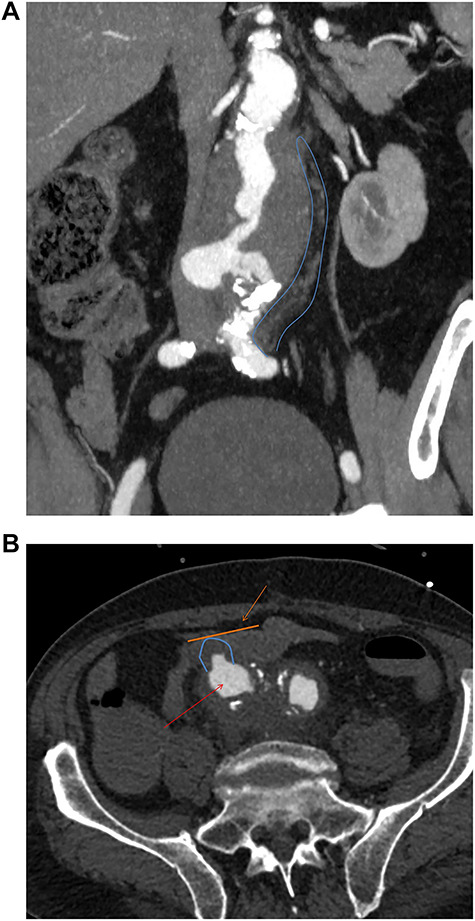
(a) Preoperative coronal thick slice of a CT with IV contrast in the arterial phase shows aneurysmal aorta with normal calibre common iliac arteries; however, inflammatory change around the aortic aneurysm is seen extending into the proximal iliac bifurcation (blue outline); (b) axial slice of a CT with IV contrast in the arterial phase shows aneurysmal common iliac arteries (red arrow), a clear saccular bulge (blue outline) into an adhered loop of small bowel (orange outline and arrow); the site of the fistulation.
